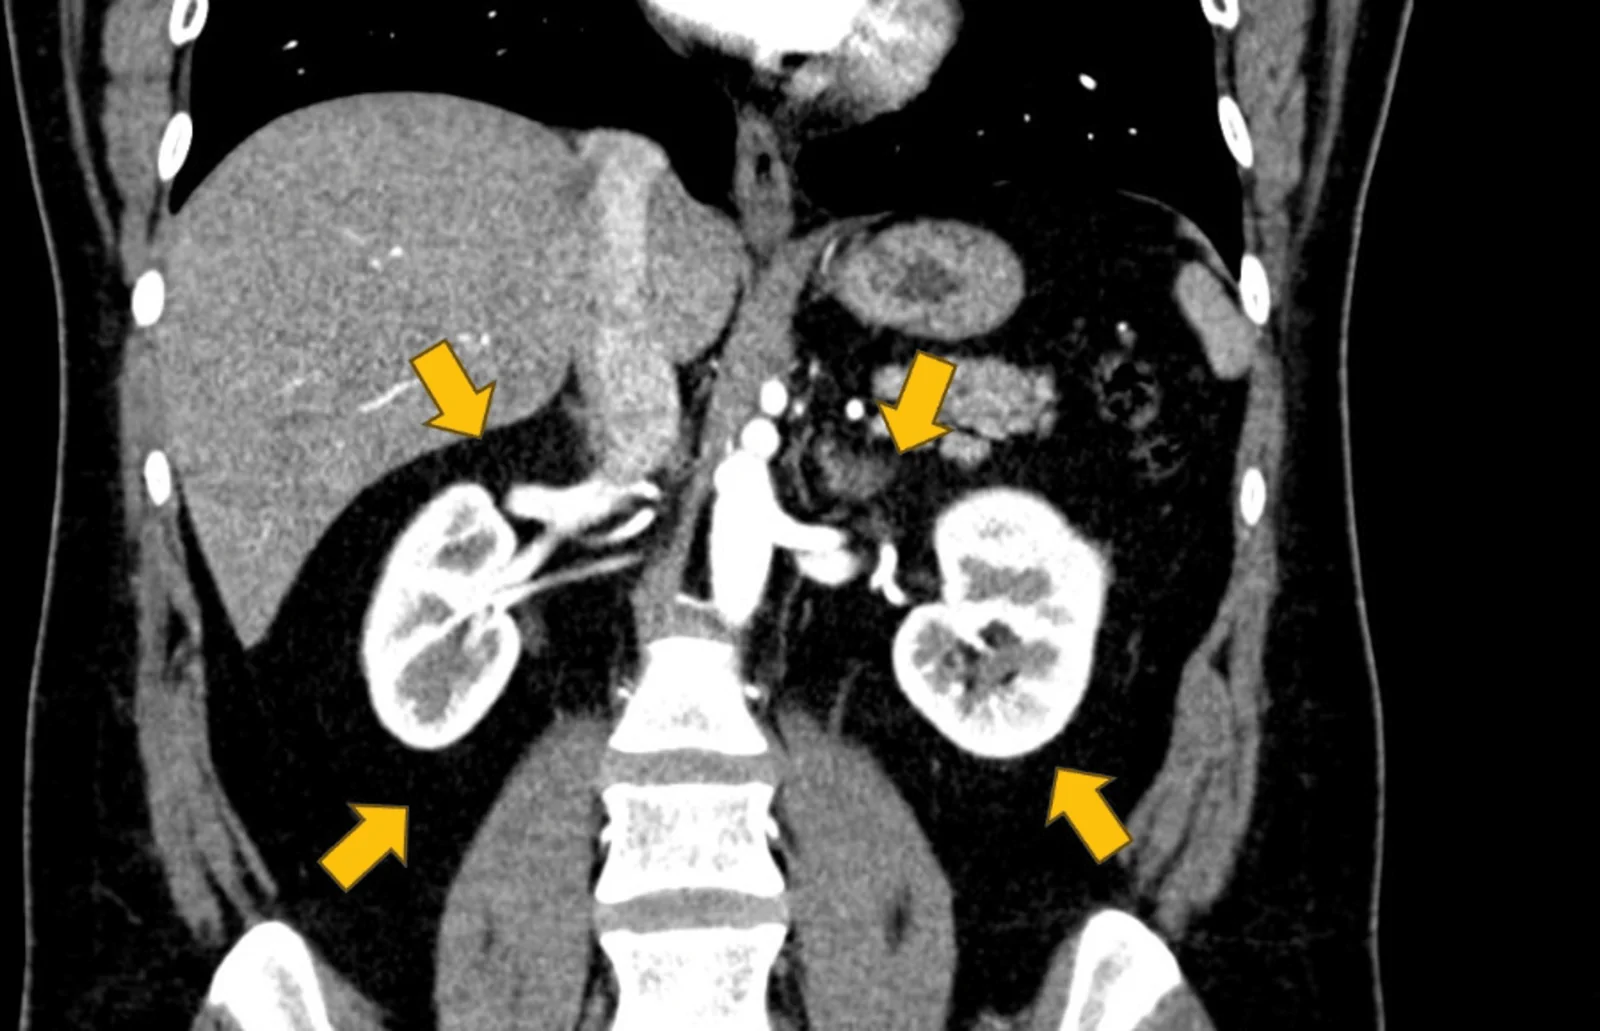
Donor computed tomography angiography demonstrating favorable renal vascular anatomy for living kidney donation. Coronal CT angiography of the abdomen and pelvis demonstrates symmetric bilateral renal enhancement and renal volumes, with the right kidney measuring 209 cc and the left kidney measuring 210 cc. The yellow arrows highlight the bilateral kidneys and renal hilar regions used to assess donor renal anatomy. Preoperative imaging demonstrated a single left renal artery, two right renal arteries including an accessory lower pole artery, and single renal veins bilaterally, supporting favorable vascular anatomy for donor nephrectomy planning.
